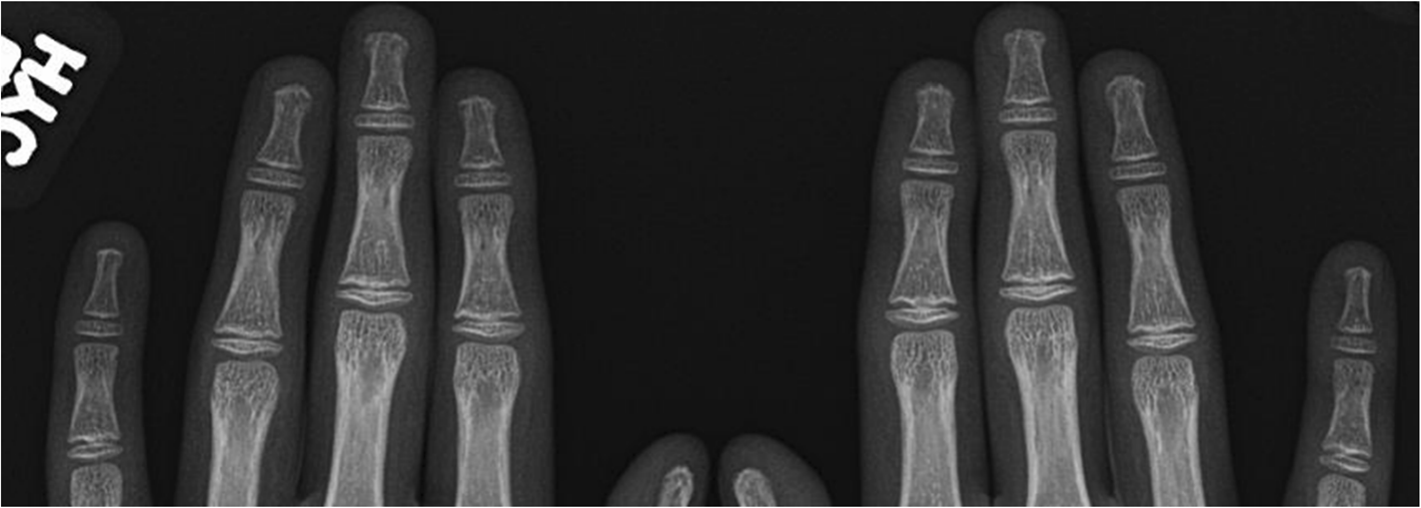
Frontal radiographs of the hands in a 13 year old male patient with systemic sclerosis show tuft resorption consistent with acro-osteolysis. No soft tissue calcifications present
